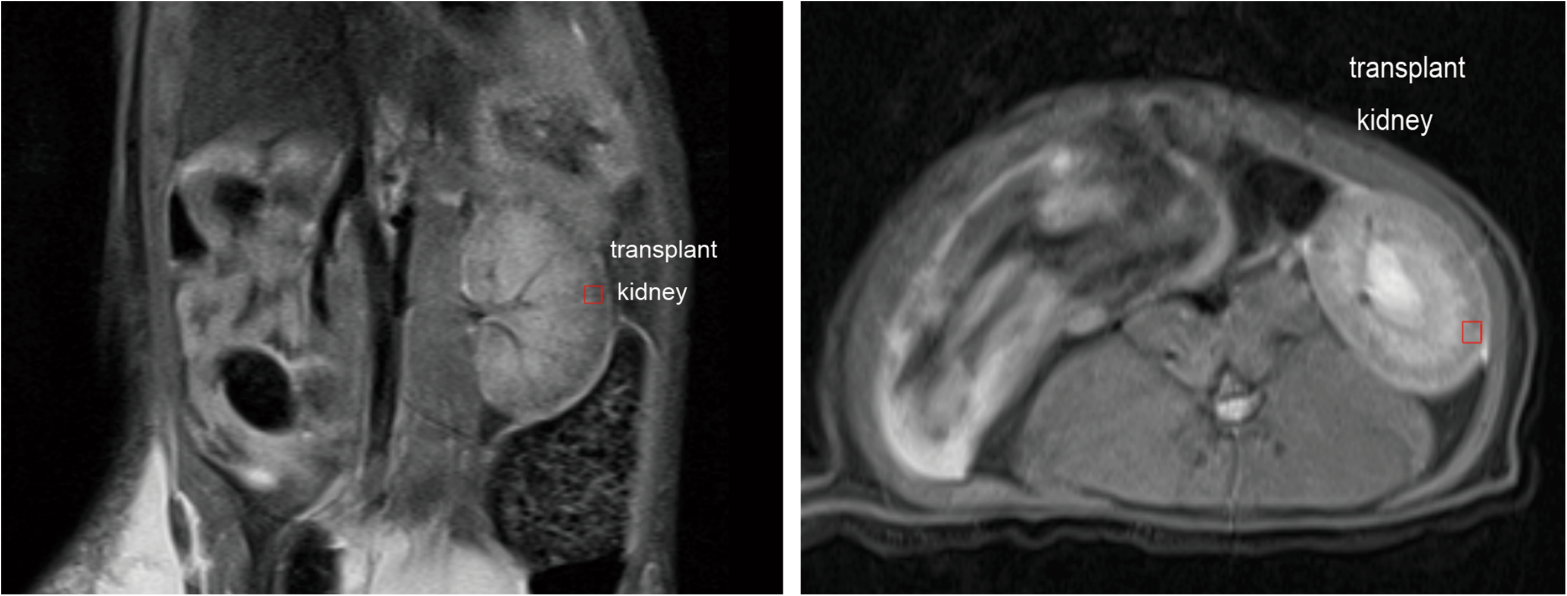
Locations of the transplanted kidneys and selection of the ROI in coronal and axial T2-weighted MRI. The transplanted kidneys were located in the original location and showed relative fixation. The regions of interest were chosen from the renal cortex randomly after avoiding blood vessels, the renal pelvis and the calyx
